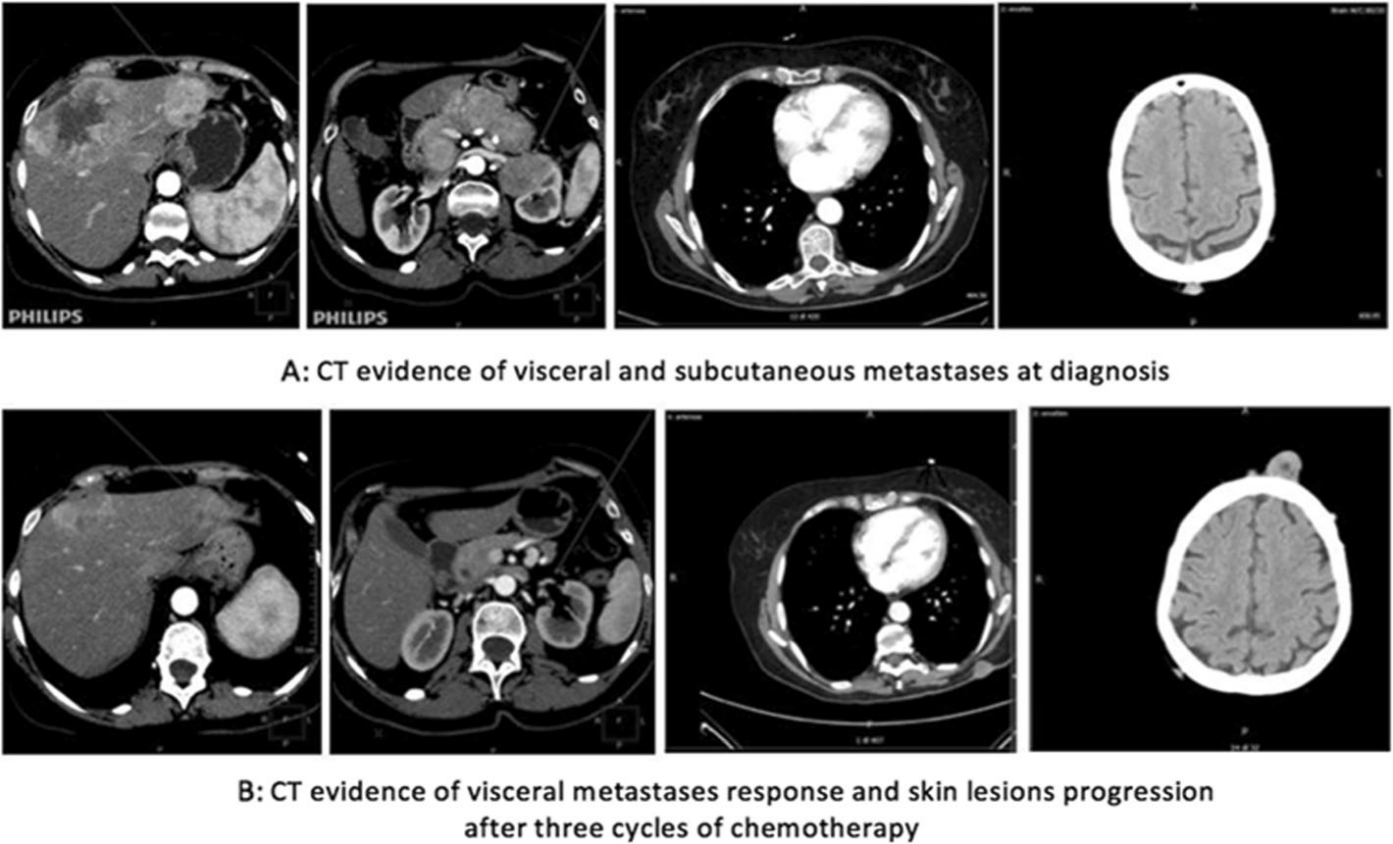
Changes in visceral and skin metastases detected by CT images
